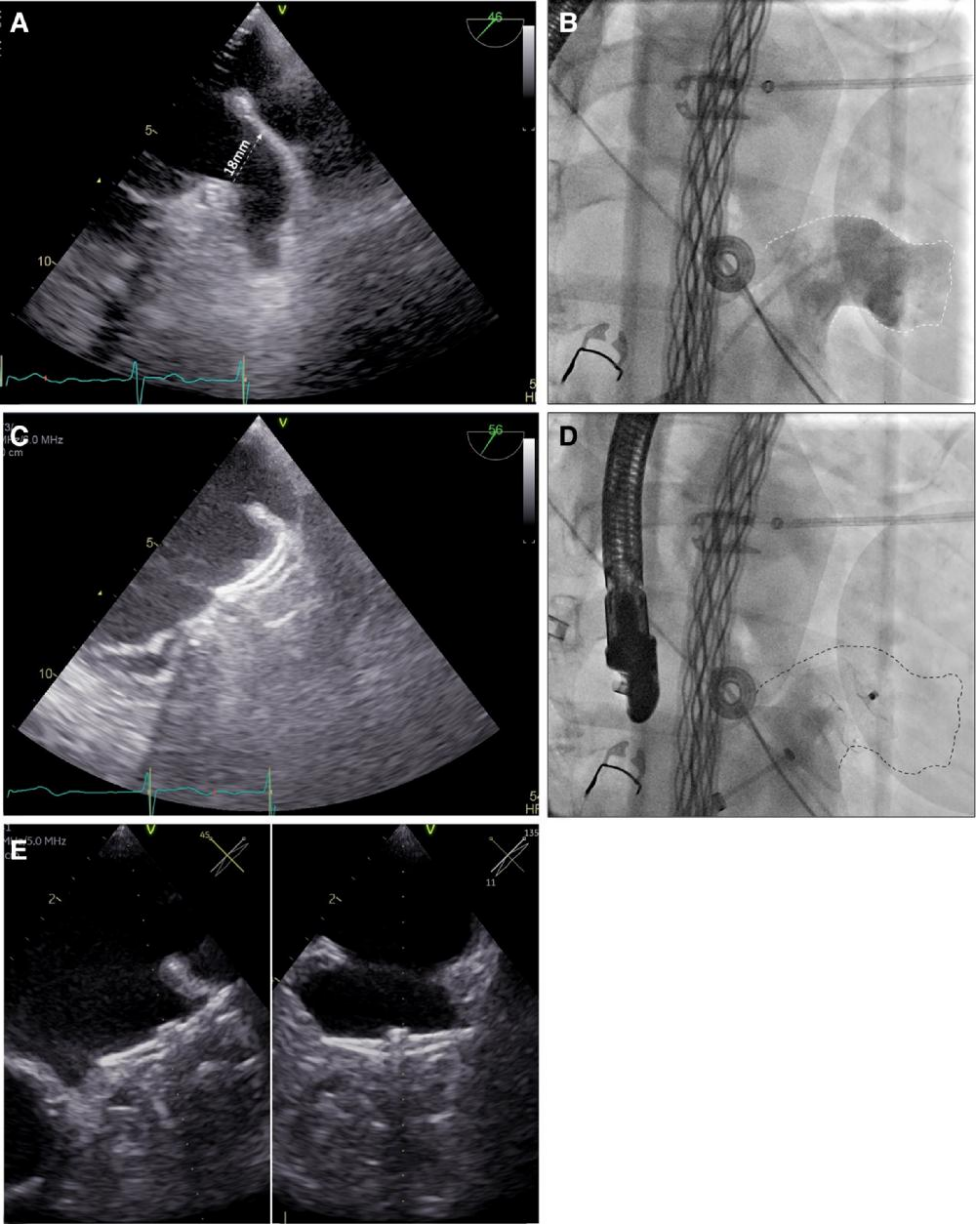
(*A*) TEE showing the LAA after ablation with a landing zone diameter of 18 mm (white arrow). (*B*) Fluoroscopic image in RAO 30°/cranial 15° projection with contrast angiography confirming a landing zone of 18 mm. (*C*) TEE demonstrating complete sealing of the LAA and a device compression of 21 mm. (*D*) Fluoroscopic RAO 30°/cranial 15° view with angiography confirming complete LAA occlusion with no residual contrast flow into the appendage. (*E*) Follow-up imaging after 6 weeks showing complete sealing of the LAA without residual leakage. LAA, left atrial appendage; RAO, right anterior oblique; TEE, transesophageal echocardiography.
